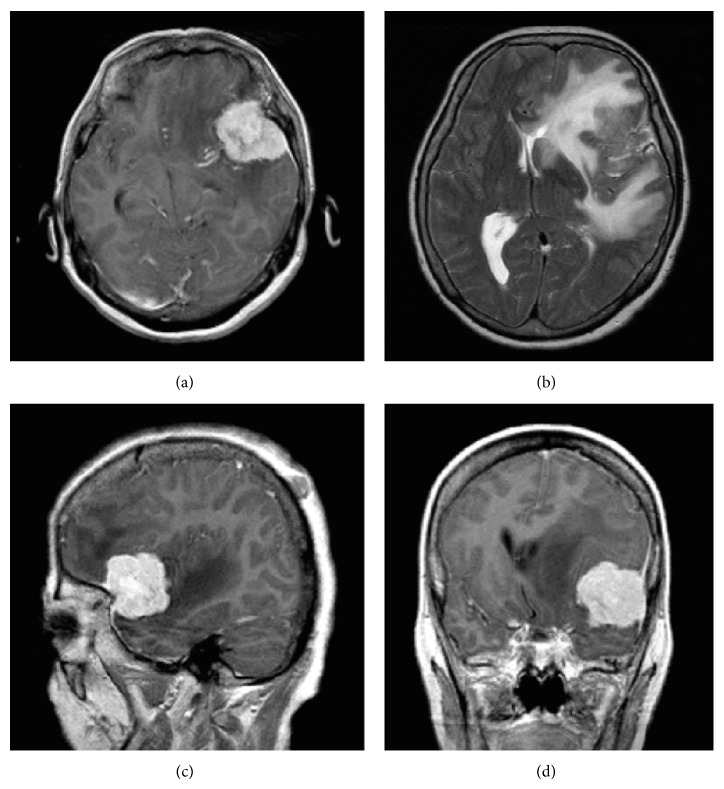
(a)–(d) MRI scans show transitional meningioma (G1) with peritumoral brain edema III°.
